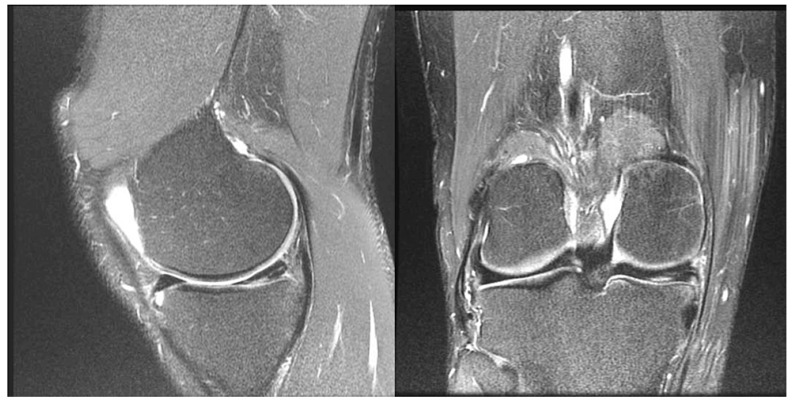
Example of a true negative. Predicted to be non-repairable by reviewers 2 and 3, but repairable by reviewer 1. Found non-repairable during arthroscopy. Sagittal (left) and coronal (right) proton density fat saturation MRI with a horizontal increased signal at the undersurface of the posterior horn of the medial meniscus from meniscal tearing. The tear is of less than 50% of the meniscal thickness.
